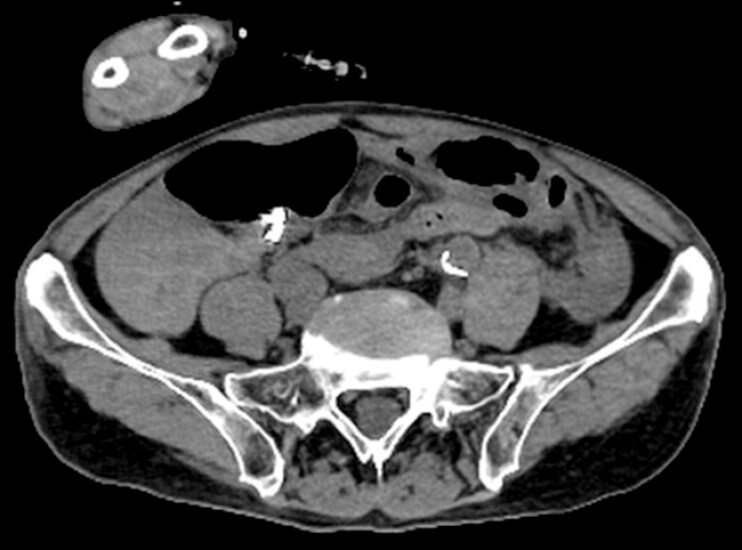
Computed tomography image 13 days after resection.
